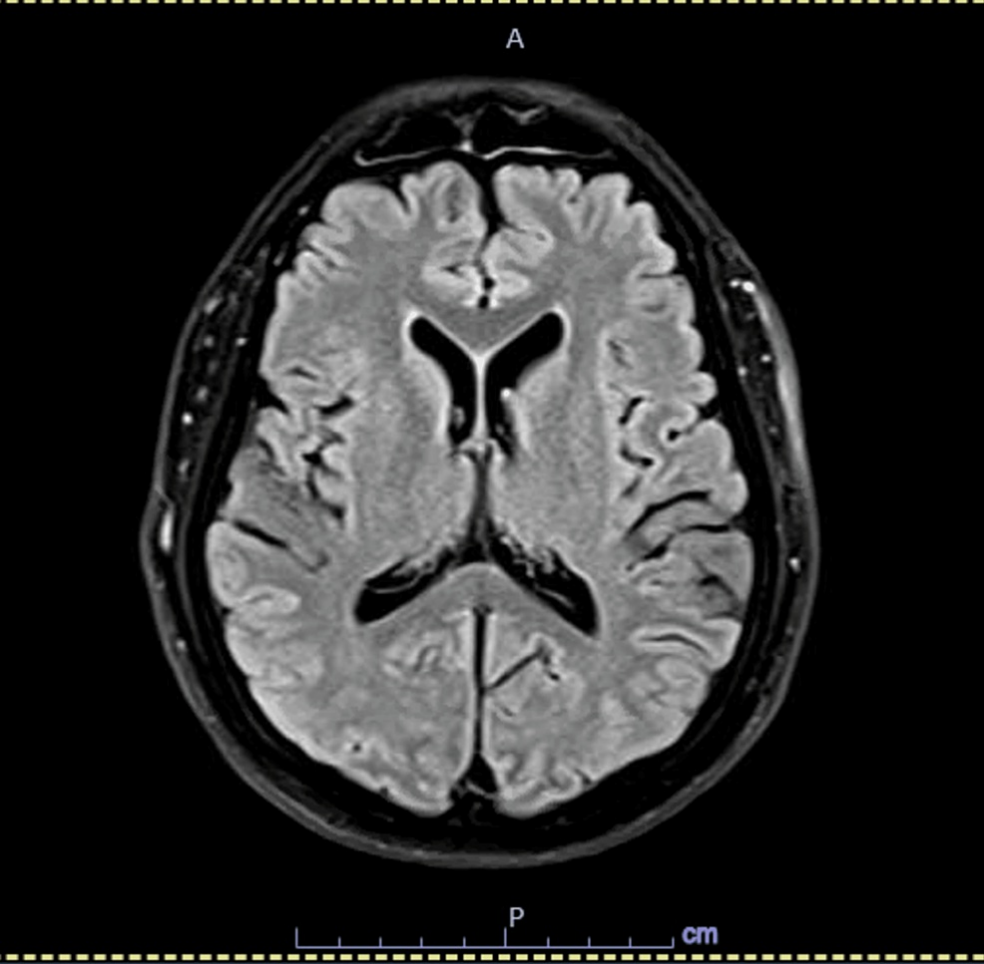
MRI Brain Magnetic resonance imaging (MRI) fluid attenuation inversion recovery (FLAIR) sequence of the brain with no reported obvious pathology.
